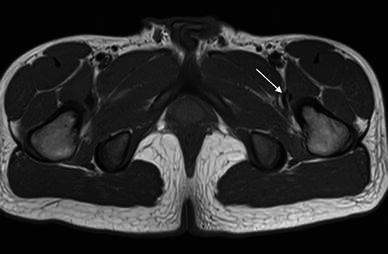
Transverse T1-weighted image in the same patient, showing the bifid iliopsoas tendon
